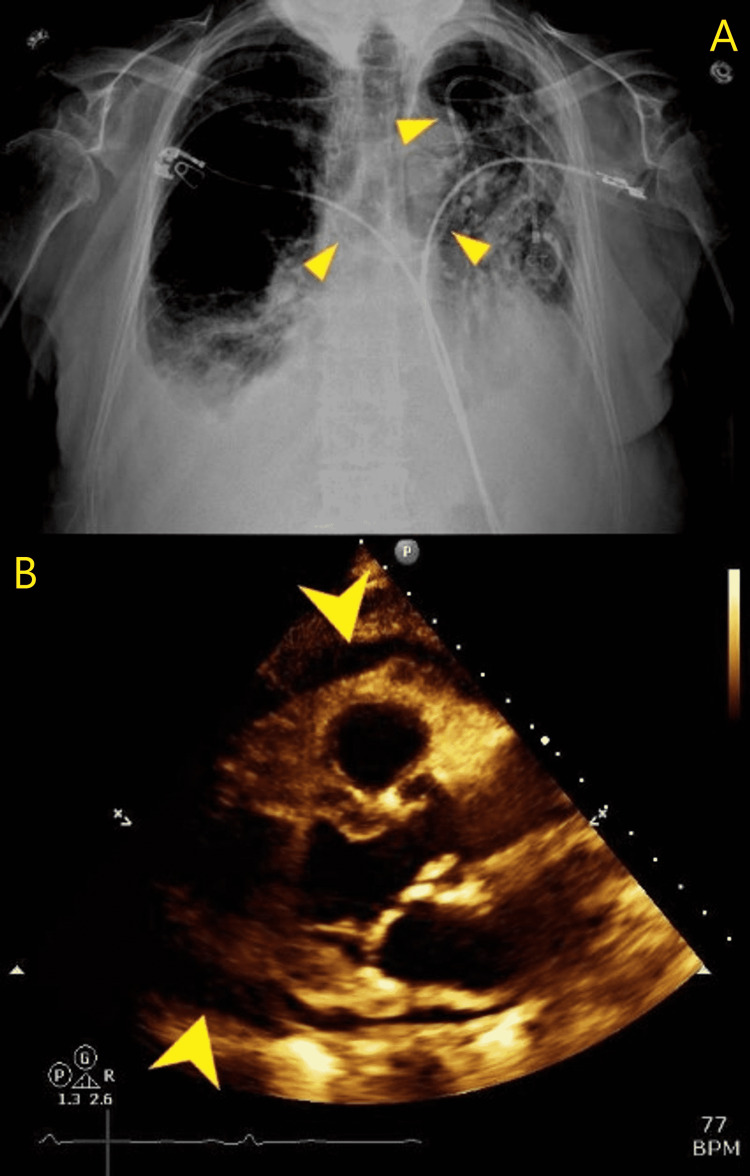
(A) Chest X-ray (AP view) - chest X-ray demonstrating bilateral interstitial infiltrates and the malpositioned tip of the mediport in the right mediastinum (yellow triangles). (B) Long axis echocardiogram view demonstrating pericardial effusion (yellow arrowheads). No tamponade physiology is seen. AP, anteroposterior
